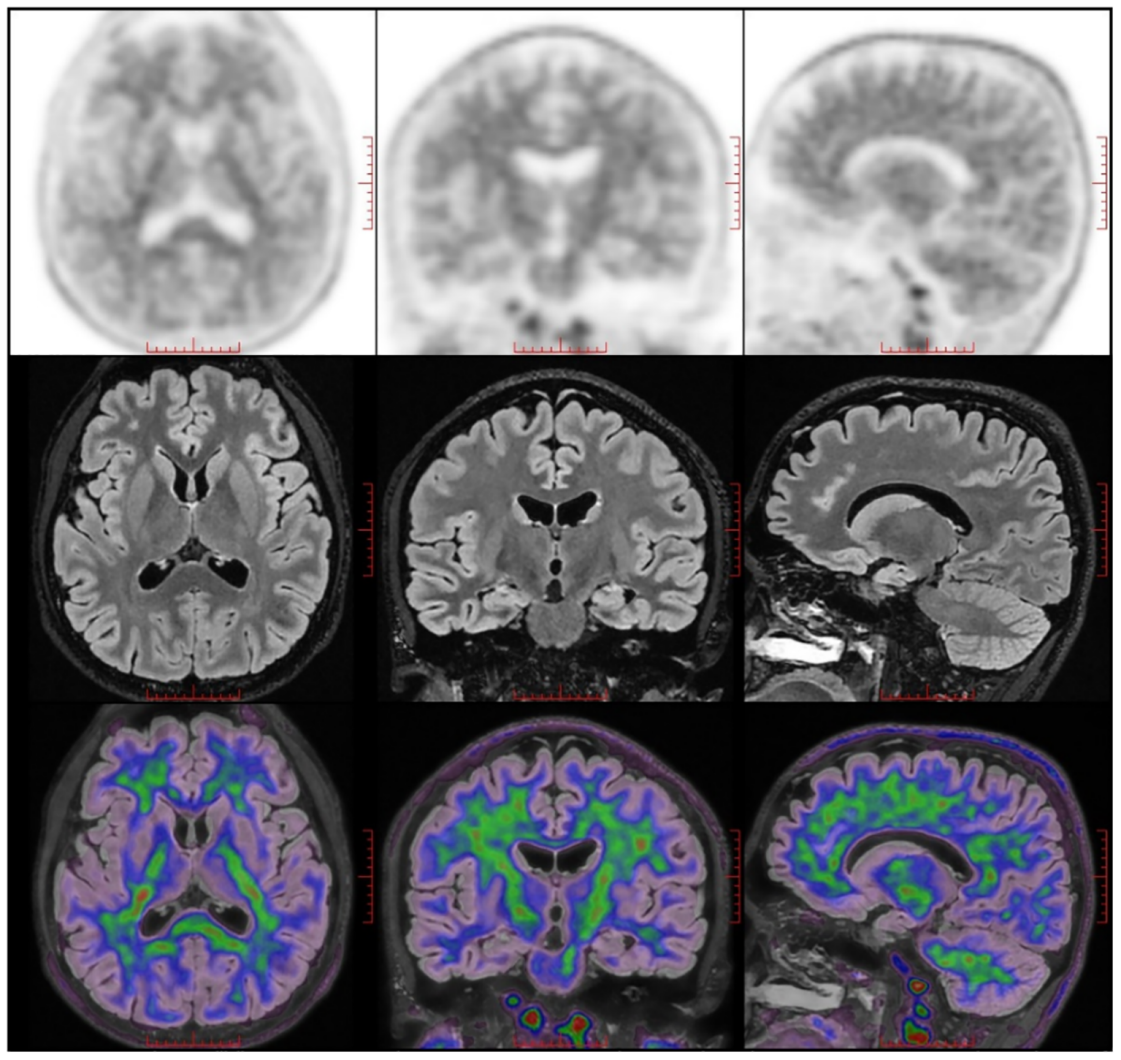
The PET/MRI images of the patient. ^18^F-AV45 PET/MRI showed no significant cortical Aβ deposition.
